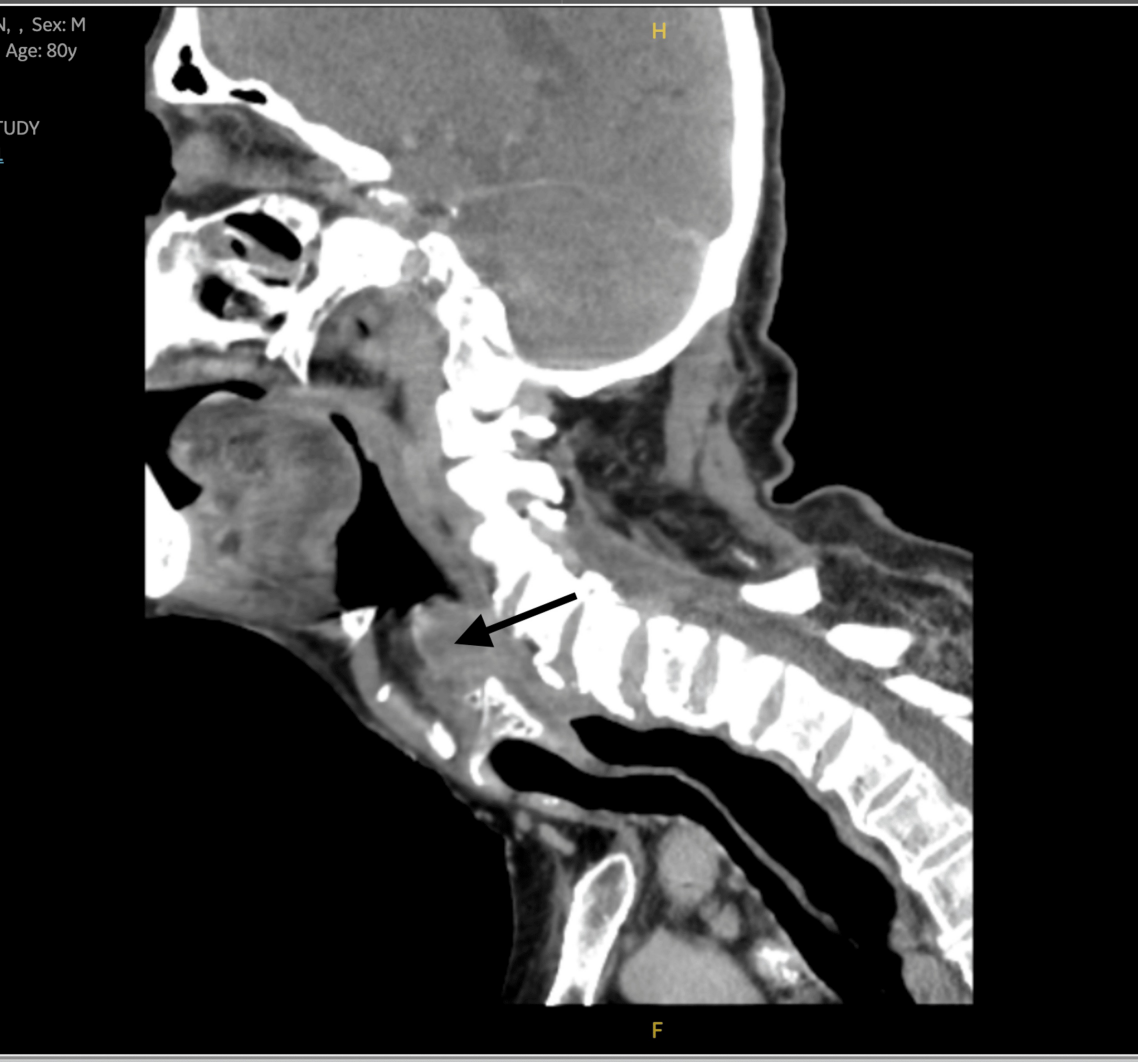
Sagittal view of the CT scan showing a hypopharyngeal cyst (black arrow) compressing the upper aerodigestive tract
